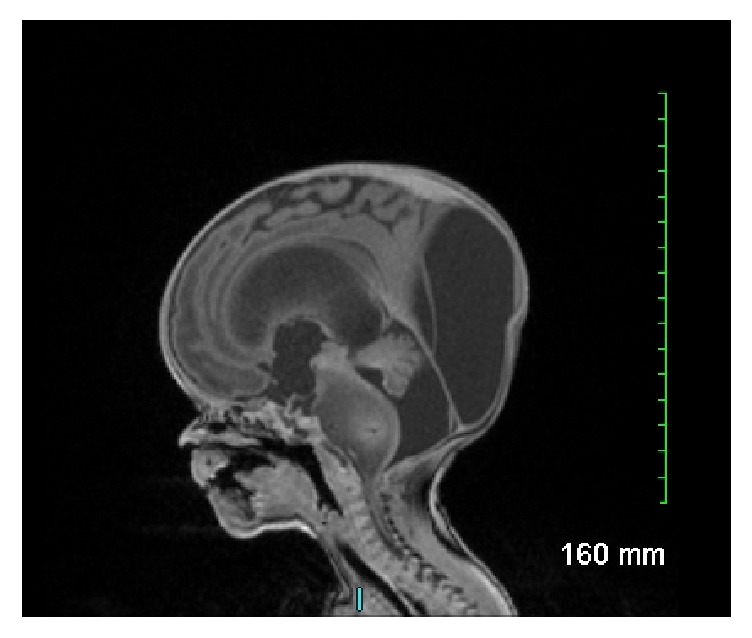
MRI showing an expansile, poorly demarcated mass in the pons with minimal heterogenous enhancement and severe communicating hydrocephalus.
